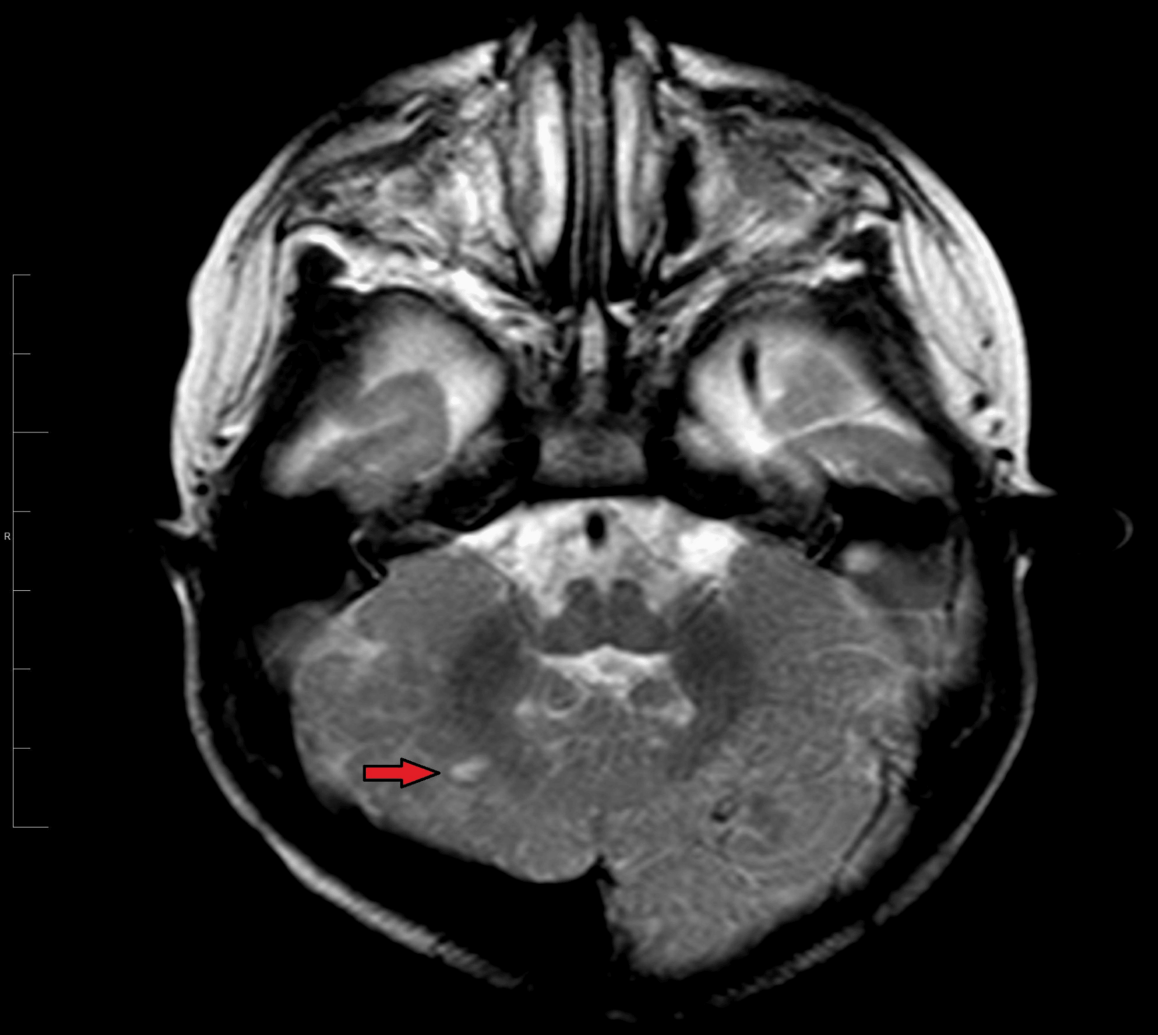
An axial section of the T2 brain MRI scan of case 1 showing abnormal cerebellar foliation and a right parenchymal cerebellar cyst. The red arrow is pointing toward the mentioned parenchymal cerebellar cyst.
